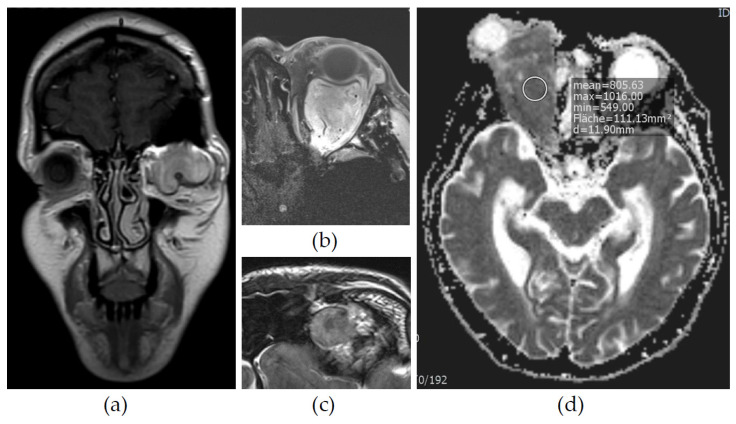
Four years later, the same patient presented with an increasing tumor growth with compressive behavior (a). Like the original tumor before (for CT scans, see Figure 2b–d), the recurrent tumor mass showed a signal intensity void (b). This phenomenon was found in 6 other tumors and is considered a radiomorphological correlate of intratumoral vessels. A chemical shift artefact was present in 3 of 9 tumors (c). Low ADC values (<1000 × 10^−6^ mm^2^/s) as a correlate of diffusion restriction was found in around the half of the tumors (4/9) (d). (a): 37-year-old female, contrast media T1 SE coronal MRI, arrow indicates optic nerve. (b): 33-year-old female, axial T1 TSE FS axial MRI, arrows indicate signal intensity void. (c): 27-year-old male, axial T2 TSE MRI, arrows indicate chemical shift artefact. (d): 87-year-old female, MRI, ADC, ROI indicates reduced mean ADC.
